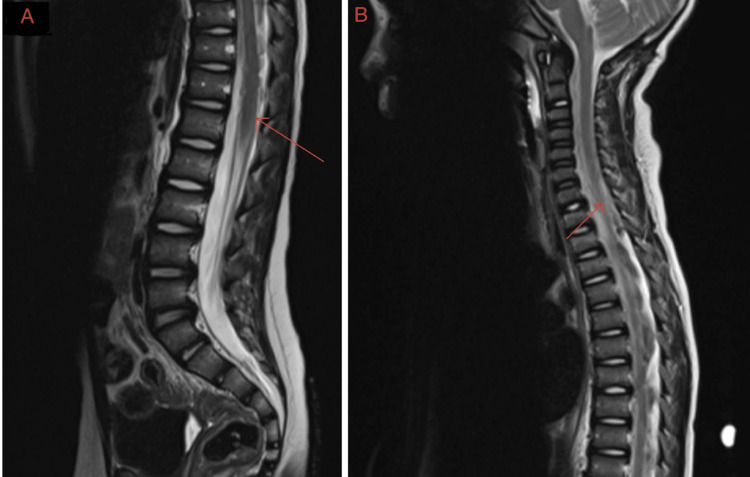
Sagittal MRI demonstrating leptomeningeal dissemination (red arrows) The image shows recurrence via leptomeningeal dissemination throughout the spinal column (red arrows). Imaging was warranted due to a new onset of vomiting and ataxia. MRI: Magnetic resonance imaging.
